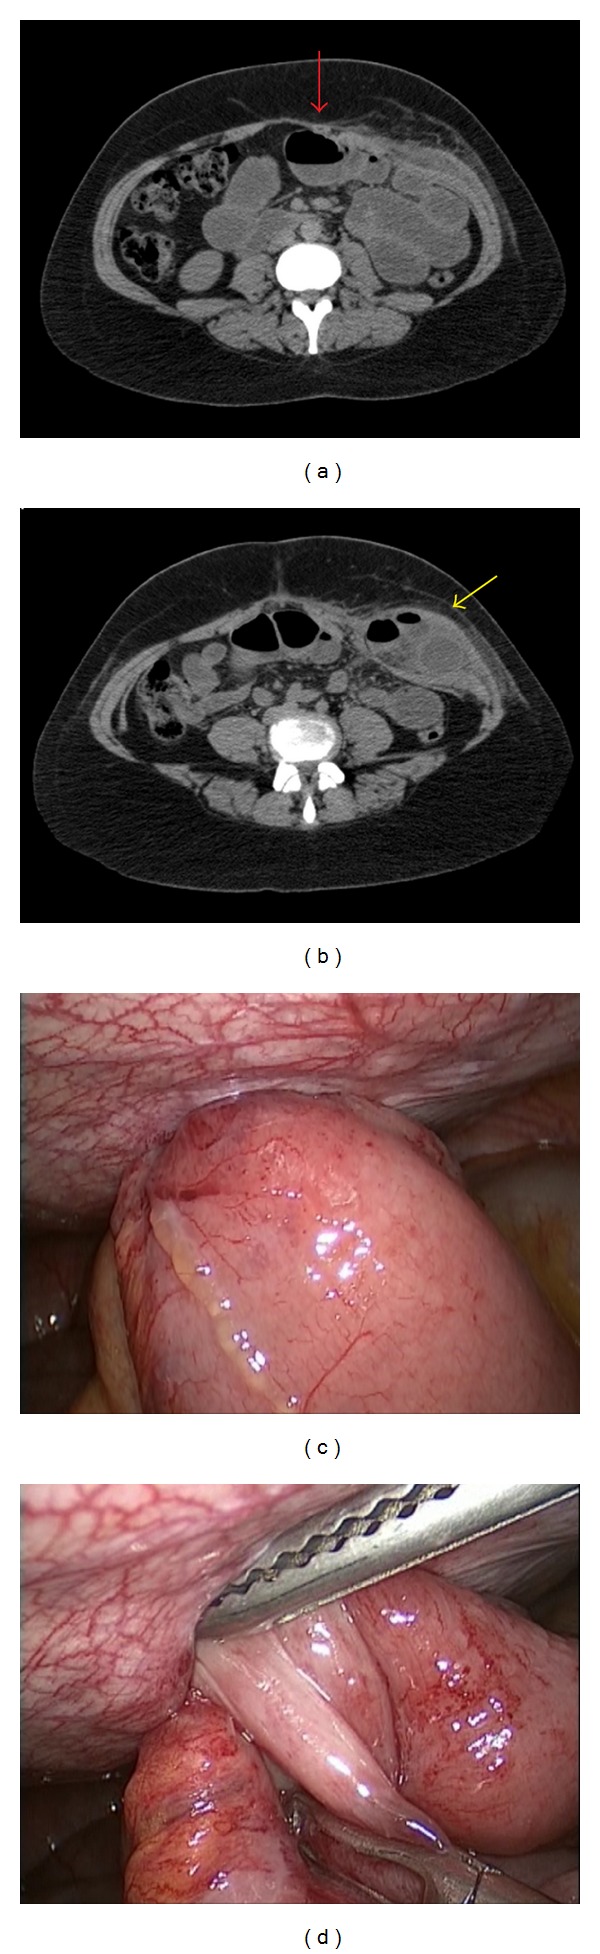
(a and b) Computed tomography of the abdomen and pelvis demonstrating the presence of small bowel loops herniated through the abdominal wall at the left iliac fossa (yellow arrow), with dilated proximal small bowel segments (red arrow), suggestive of incarcerated incisional hernia. (c and d) Laparoscopic surgery confirming the presence of an incarcerated herniation of the small bowel.
